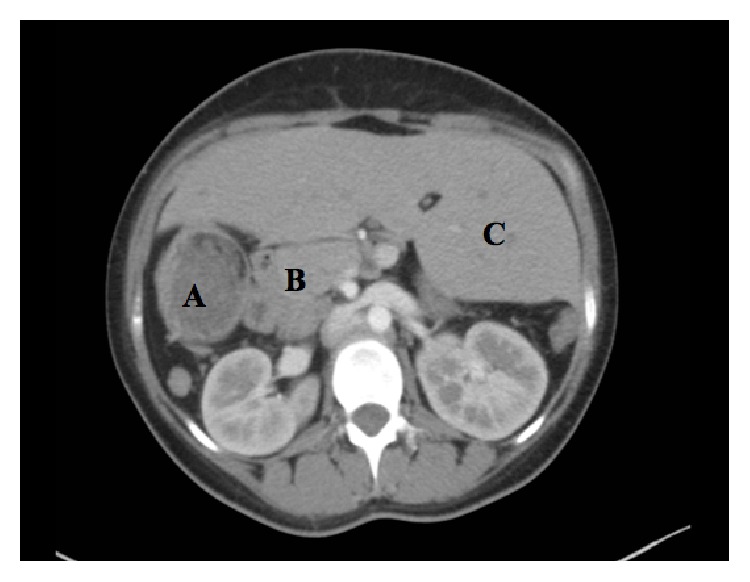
CT scan of the chest with contrast demonstrating situs ambiguous: (A) a right-sided stomach, (B) multiple splenules on the right, and (C) the liver in the midline occupying both right and left portions of the abdomen.
